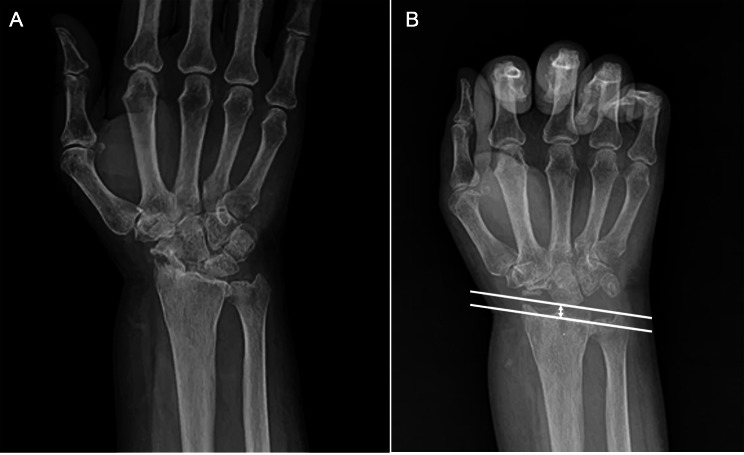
Simple radiography of 67-year-old female who underwent proximal row carpectomy with interposition arthroplasty utilizing both a capsular flap and acellular human dermal matrix (A) Radiographic images taken before the surgery on the patient’s wrist revealed significant arthritic alterations in the lunocapitate and radiolunate joints, along with a collapsed scaphoid. (B) On radiographic examination one year post-surgery, it was observed that the radiocarpal distance was well maintained
